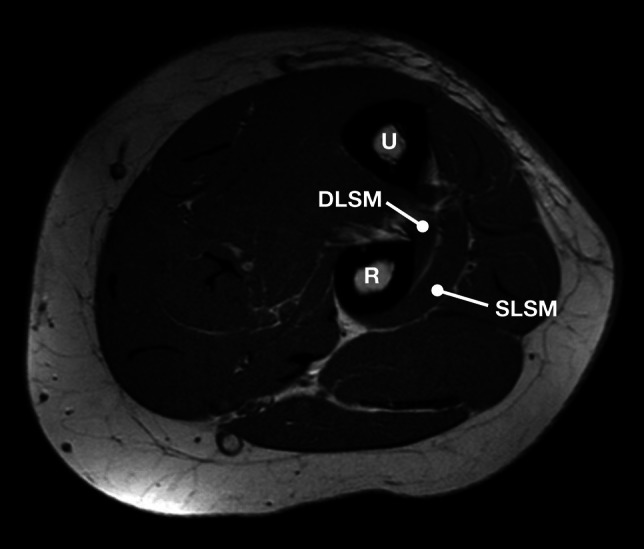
Left elbow MRI without the bare area. DLSM = deep layer of the supinator muscle; R = radius; SLSM = superficial layer of the supinator muscle; U = ulna
